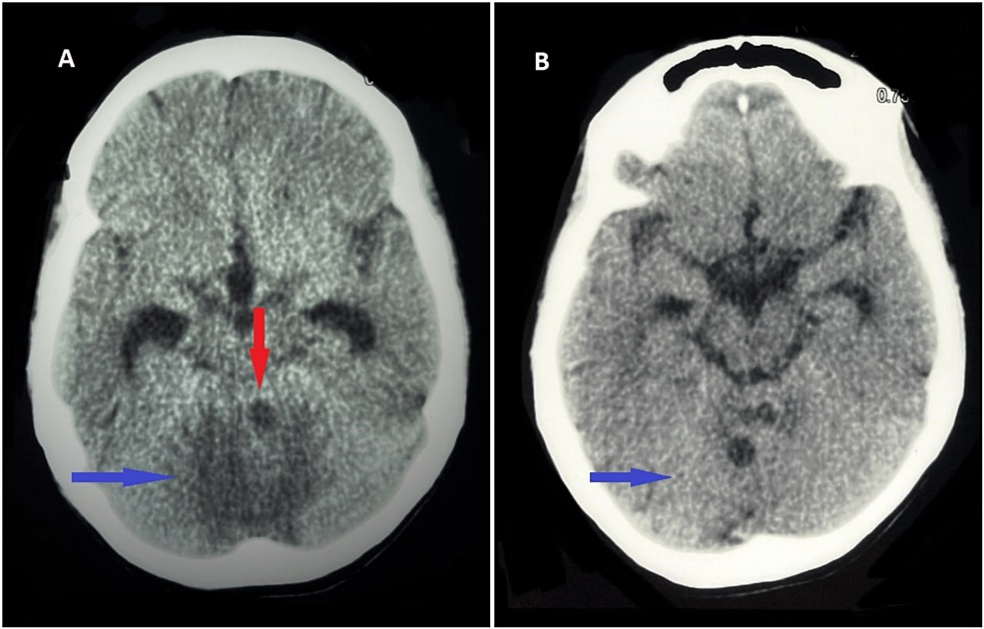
CT scan of the brain (plain) A: Axial view showing hypodense lesion (red arrow) in cerebellar vermis with significant perilesional edema (blue arrow). B: Axial view showing significant resolution of perifocal edema (arrow) two weeks after initiating the treatment.
